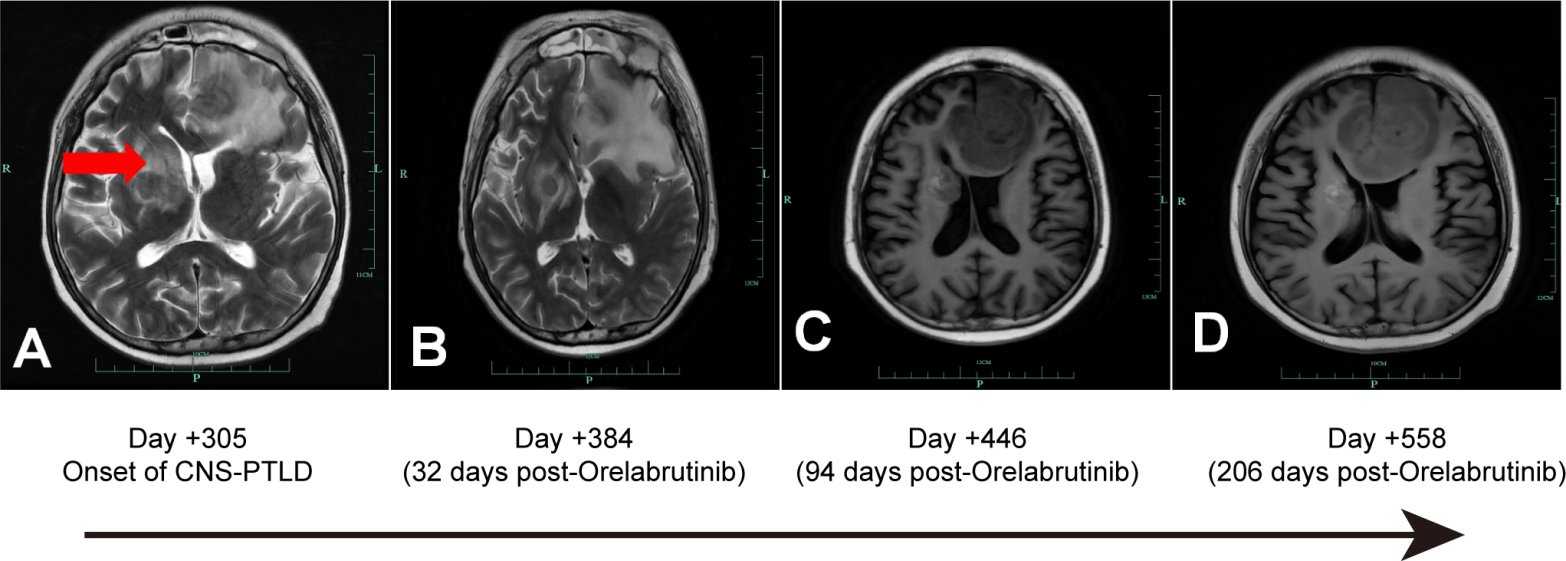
CNS-PTLD Brain MRI: (A, B) Axial T1-weighted post-gadolinium contrast-enhanced images; (C, D) Axial T2-weighted post-gadolinium contrast-enhanced images. A: Day +305 Onset of CNS-PTLD, showing a hypointense lesion in the right corpus callosum (red arrow). B: Day +384 (32 days post-Orelabrutinib): The lesion remains stable, measuring 3.14 cm at its widest point. C: Day +446 (94 days post-Orelabrutinib): The lesion and edema have decreased, with a maximum diameter of 2.30 cm. D: Day +558 (206 days post-Orelabrutinib): Further reduction in lesion size, with the maximum diameter measuring 2.0 cm.
